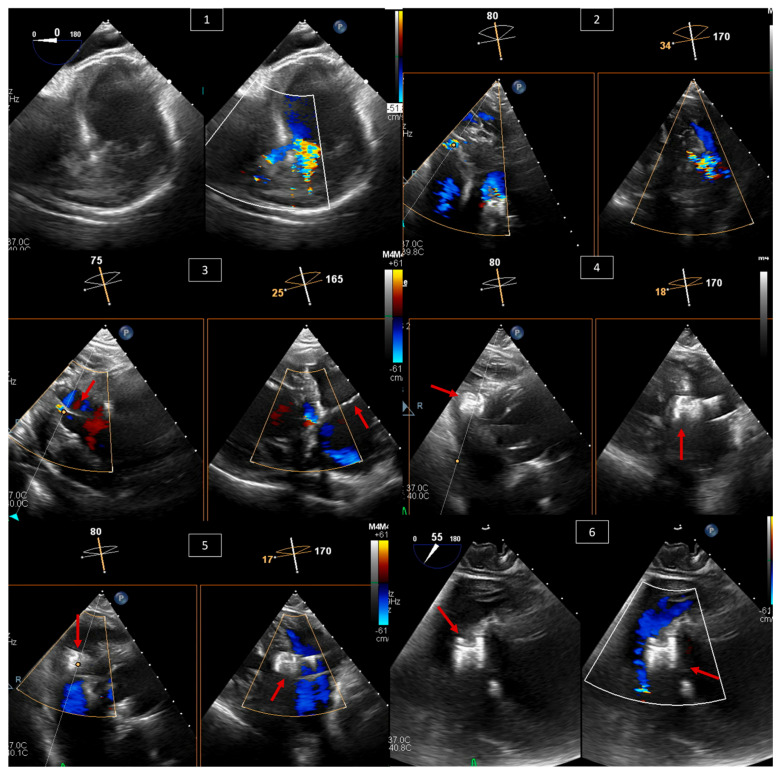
TEE of a percutaneous muscular VSD closure in a 54-year-old male with symptomatic significant left-to-right shunt. (1) Transgastric view of the muscular VSD with and without color Doppler showing left-to-right shunt. (2) X-plane on the defect with color Doppler at different angles to decide on the best angle for guiding the intervention. (3) X-plane of a transgastric view with color Doppler showing the wire (red arrow) across the VSD at 75 and 165 degrees. (4) X-plane of a transgastric view showing the VSD closure device (red arrow) in position and still attached to the wire at 80 and 170 degrees. (5) The same view from (4), now with color Doppler showing no residual left-to-right shunt. (6) Transgastric view at 55 degrees with color Doppler showing the VSD closure device (red arrow) after release in a stable position.
